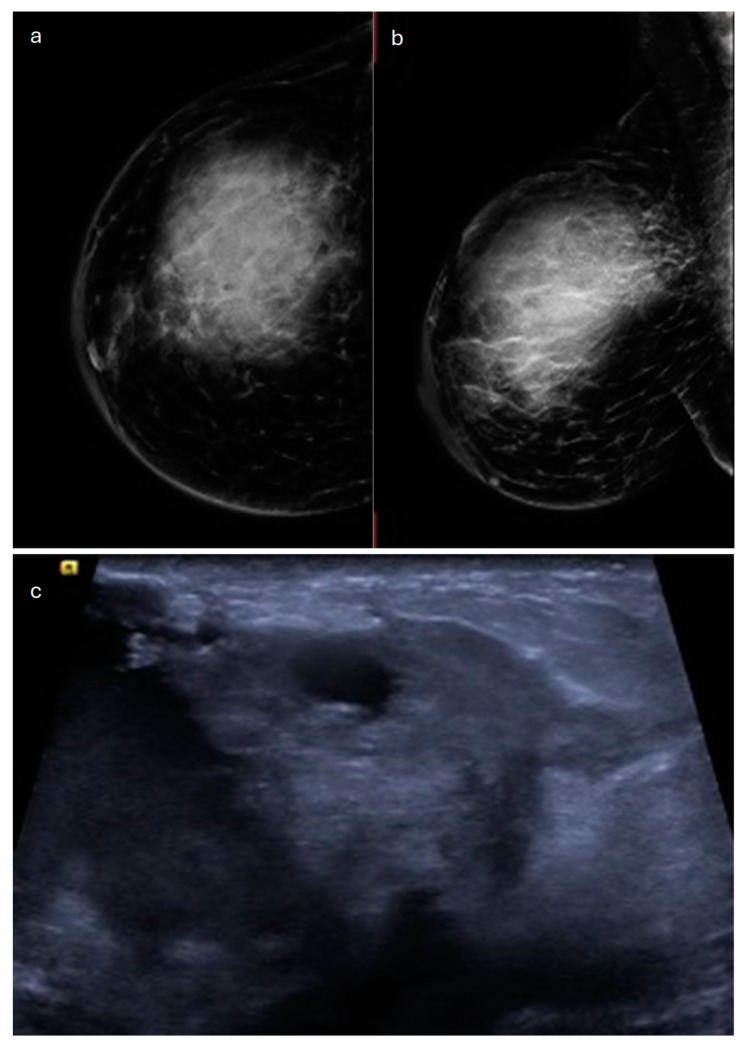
Metaplastic carcinoma in a 43-year-old patient. (a) Cranio-caudal and (b) medio-lateral oblique mammograms of the right breast show a high-density mass with indistinct margins predominantly located in the upper-outer quadrant and (c) corresponding to an iso-hypoechoic mass with partially indistinct margins and cystic components on ultrasound.
